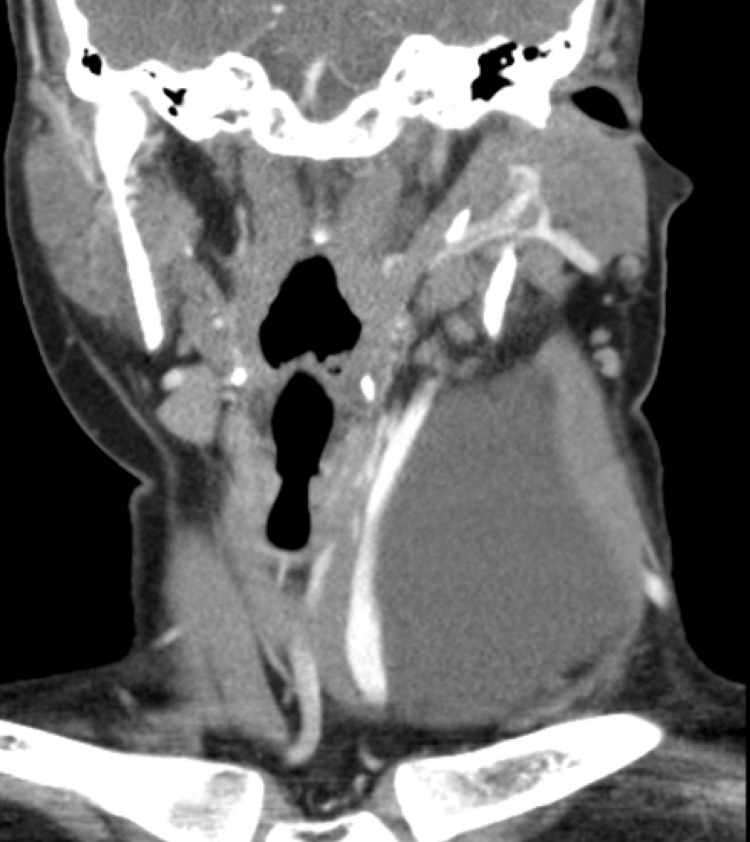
Coronal CT with contrast of BCC splaying the internal jugular vein anteriorly and medially BCC, branchial cleft cyst
